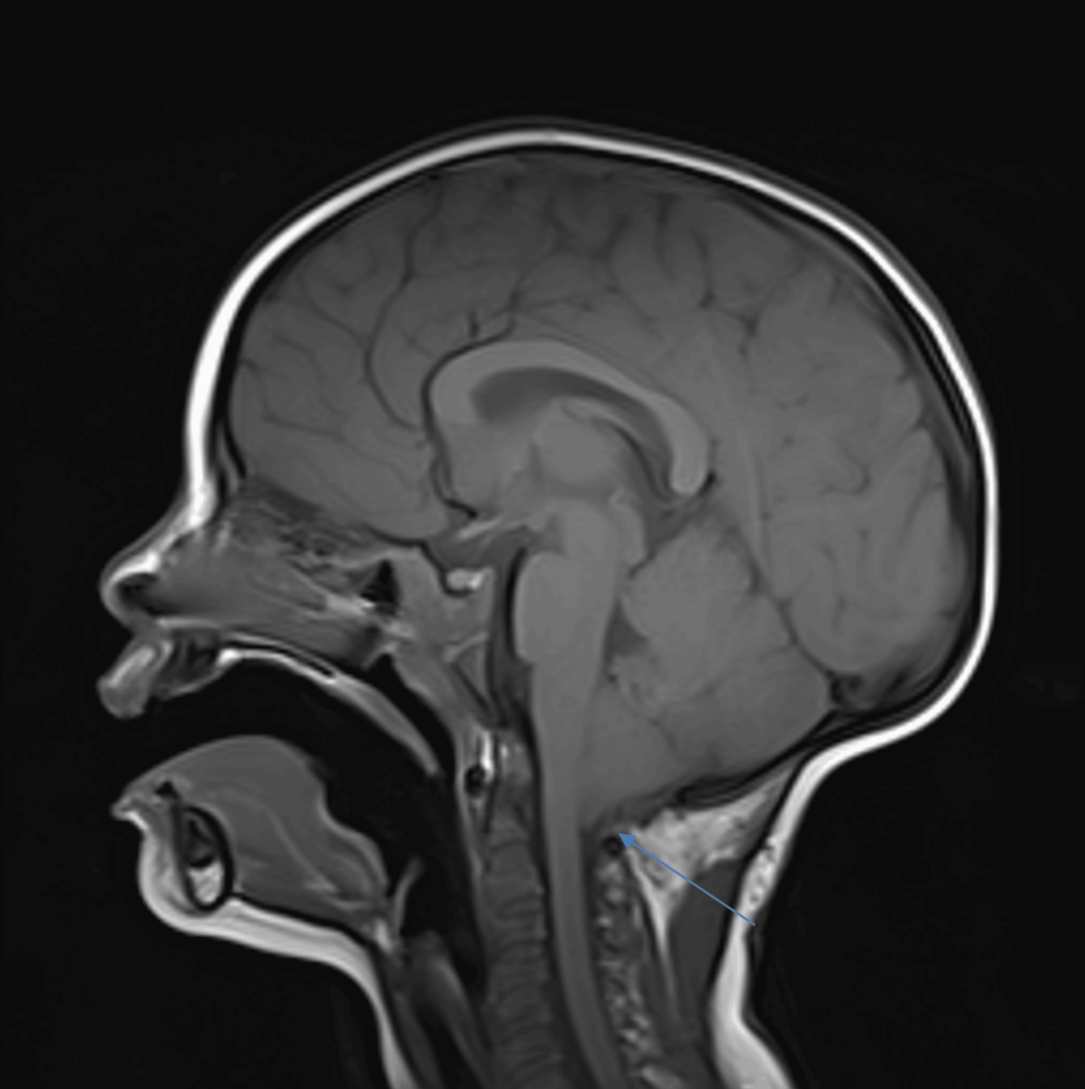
Sagittal T1 MRI imaging of the proband’s sister after surgical decompression, demonstrating improved but still present crowding and cerebellar descent.
